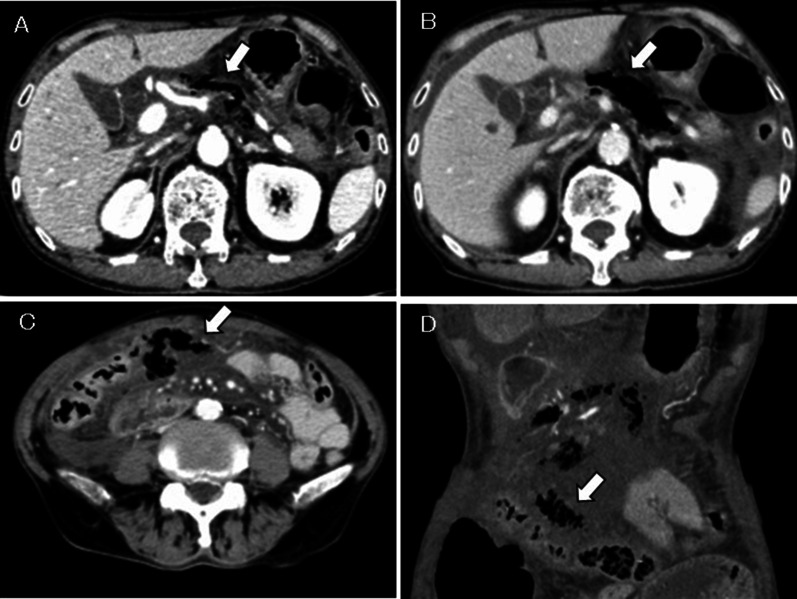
Contrast-enhanced CT scan of case 1. A Initial CT showing the emphysema around the pancreatic body (arrow). B CT performed 6 h post-admission showing a significant increase in retroperitoneal gas (arrow). C, D CT performed 6 h post-admission showing the appearance of gas in the transverse mesocolon (arrows)
